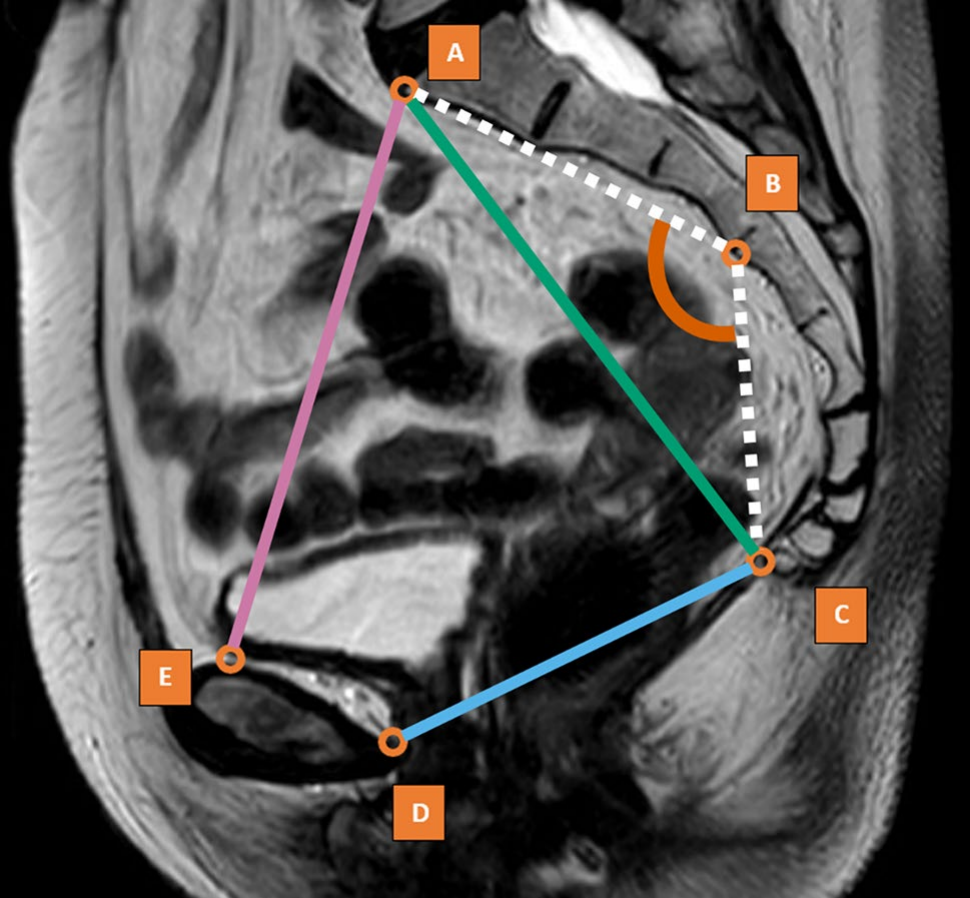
A visualization of the sagittal MRI acquisition with the five landmarks: promontorium (A), S3-vertebrae (B), coccyx (C), dorsal part of os pubis (D), and cranial part of os pubis (E). The landmarks are used to measure the pelvic inlet (purple, distance between A and E), pelvic outlet (blue, distance between C and D), pelvic depth (green, distance between A and C), and sacral angulation (orange, angle between A, B, and C) (Color figure online)
